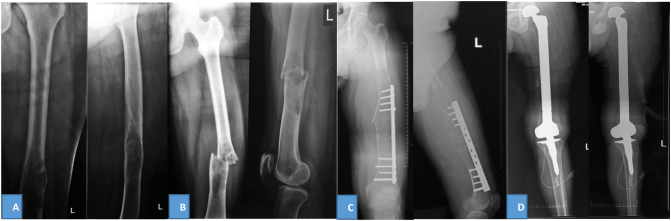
Left femur X-ray: (A) Initial X-ray of patient before fracture; (B) pathological fracture of femoral diaphysis; (C) 3 years after open reduction and internal fixation; (D) post-operative X-ray.
